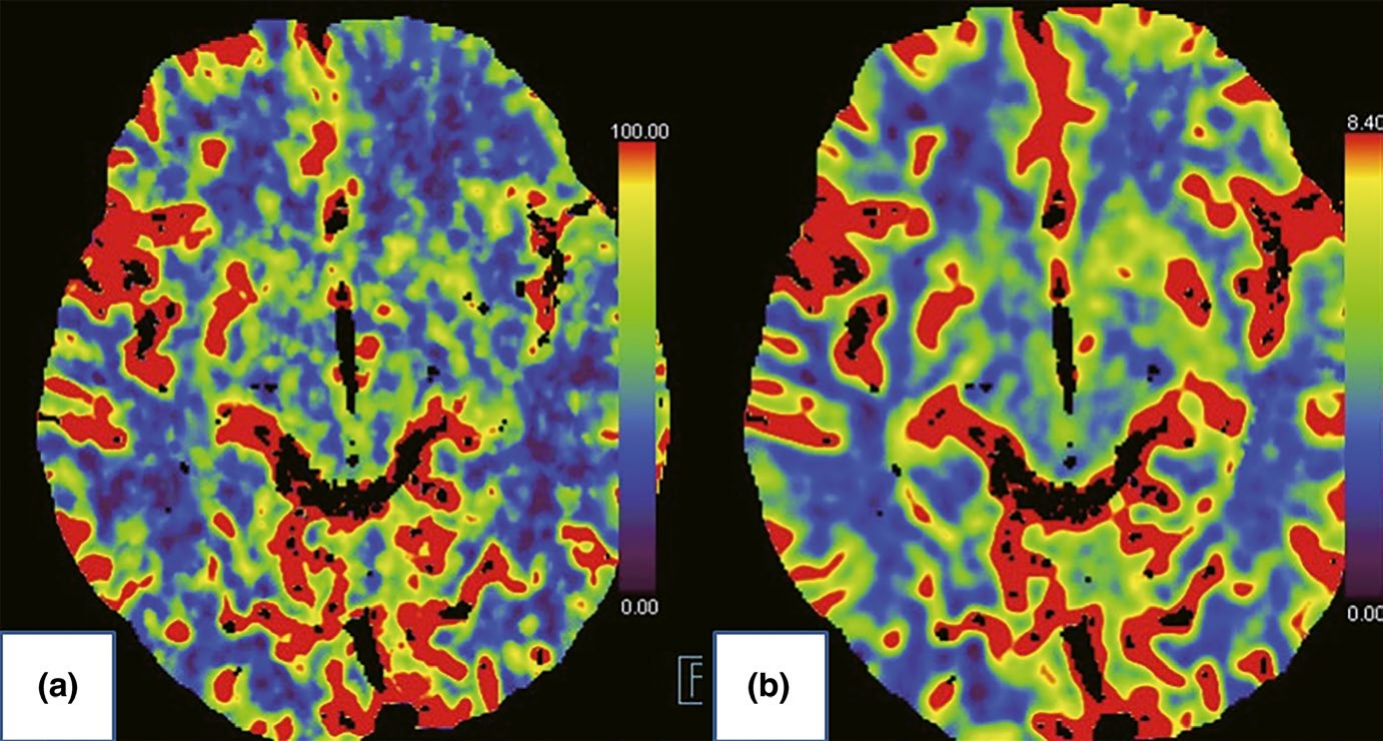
Computed tomography brain perfusion imaging. (a) Preoperative impaired left anterior frontal lobe, the left temporal lobe, and the left basal ganglia blood flow (blue). (b) Postoperative improvement
